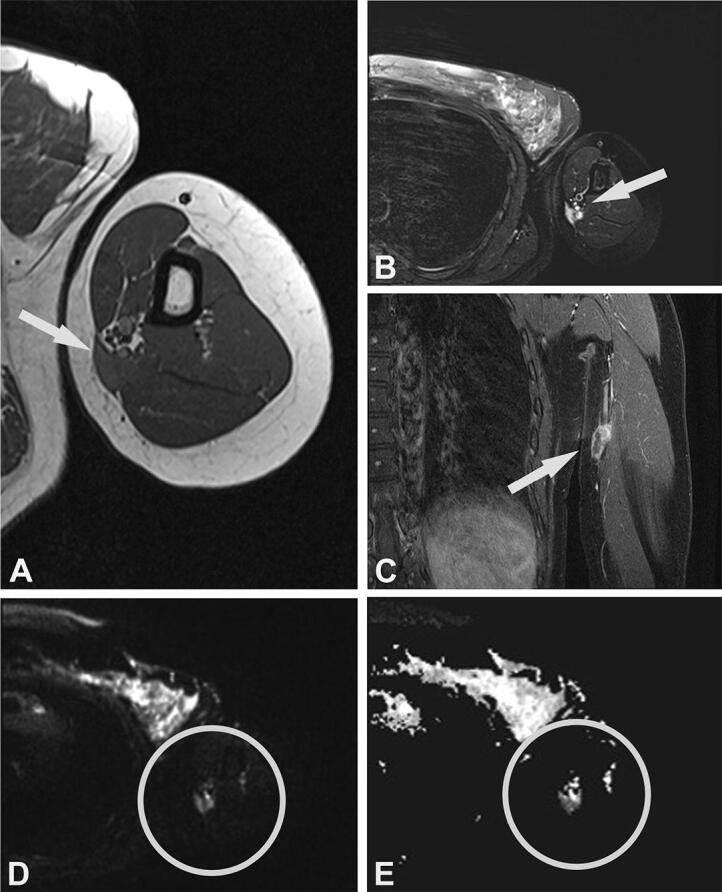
Case no. 7. A 40-year-old female with an epifascial soft tissue lesion of the upper arm. (A) On a T1-weighted image, the lesion with intermediate signal intensity and irregular margin; wide contact with the underlying fascia (arrow). (B) Homogenous high T2-weighted signal intensity without any surrounding edema (arrow). (C) Coronal T1-weighted image with fat saturation after application of Gd-contrast shows heterogeneous contrast enhancement (arrow). (D–E) DWI image (D) with corresponding (E) ADC map shows diffusion restriction due to necrotic collection (circles).
